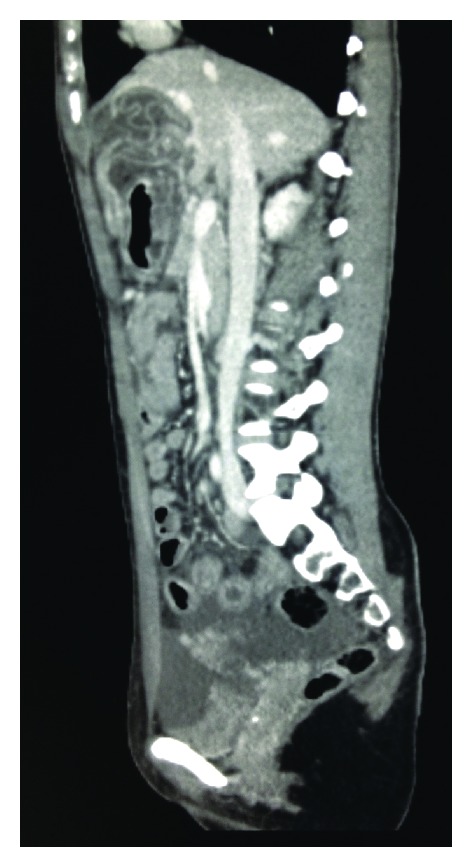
Contrast CT (Sagittal section) demonstrating the ruptured hydatid cyst within the anterior portion of the left lobe of the liver, with serpiginous strands representing the collapsed membrane. Significant free fluid is noted within the pelvis.
